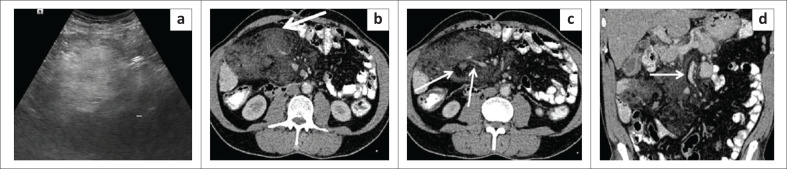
Mesenteric panniculitis. Transverse grey scale ultrasound image (a) of the abdomen in a 63-year-old male reveals diffusely echogenic mesentery with no well-defined solid mass. Axial contrast enhanced CT images of the abdomen (b, c) reveals an ill-defined inhomogenous mass of mean attenuation ~ 5.8 HU (greater than that of retroperitoneal fat). Multiple hyperdense nodules are seen within the mass and surrounding mesentery. Thin discontinuous slightly hyperattenuating soft tissue rim is seen on the anterior and posterior aspect of the mass limiting the mass from the surrounding mesentery (thick white arrow in b) – tumoural pseudocapsule sign. The mass is seen to encase superior mesenteric artery (SMA), superior mesenteric vein (SMV) and their branches with preservation of a ring of fat around mesenteric vessels and nodules (thin white arrows in c) – fat ring sign. Coronal reformatted CT image (d) reveals an ill-defined inhomogenous mass in the mesentery encasing the SMA, SMV and their branches with preservation of a rim of fat around SMA (thin white arrow – fat ring sign).
